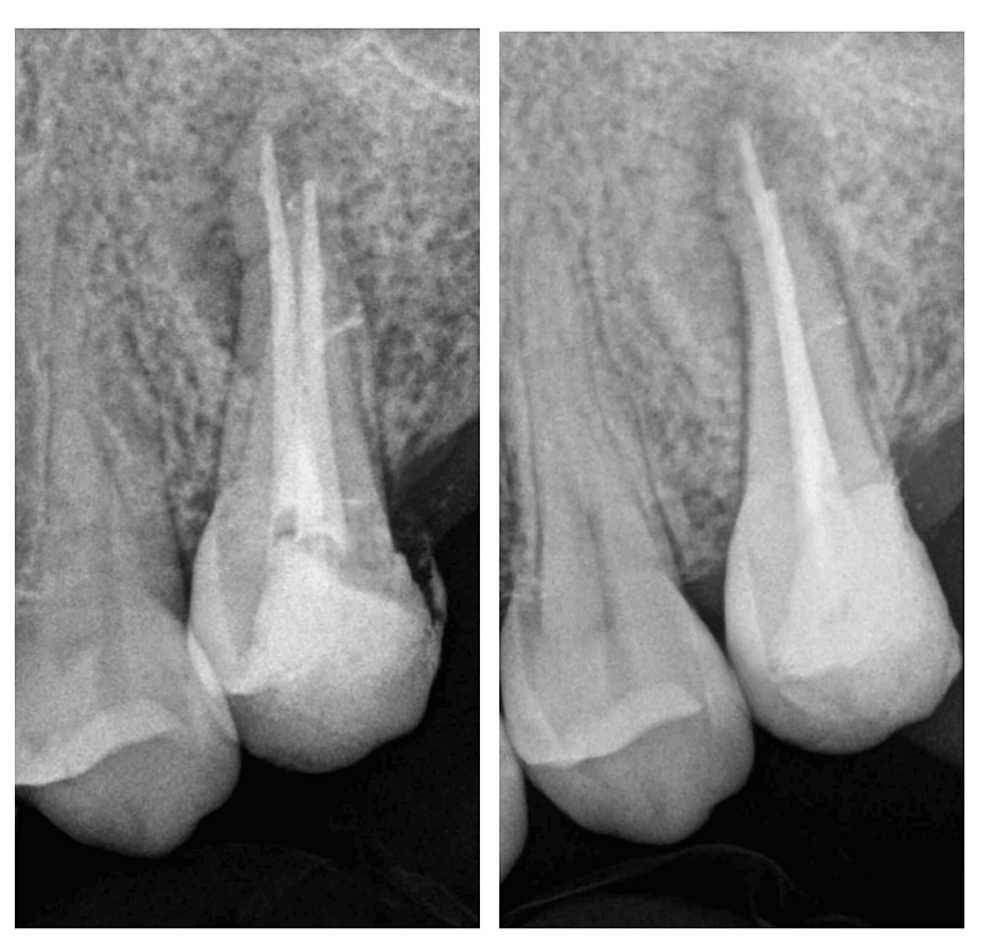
Intraoral periapical radiograph shows obturation of the root canal of tooth #25.
